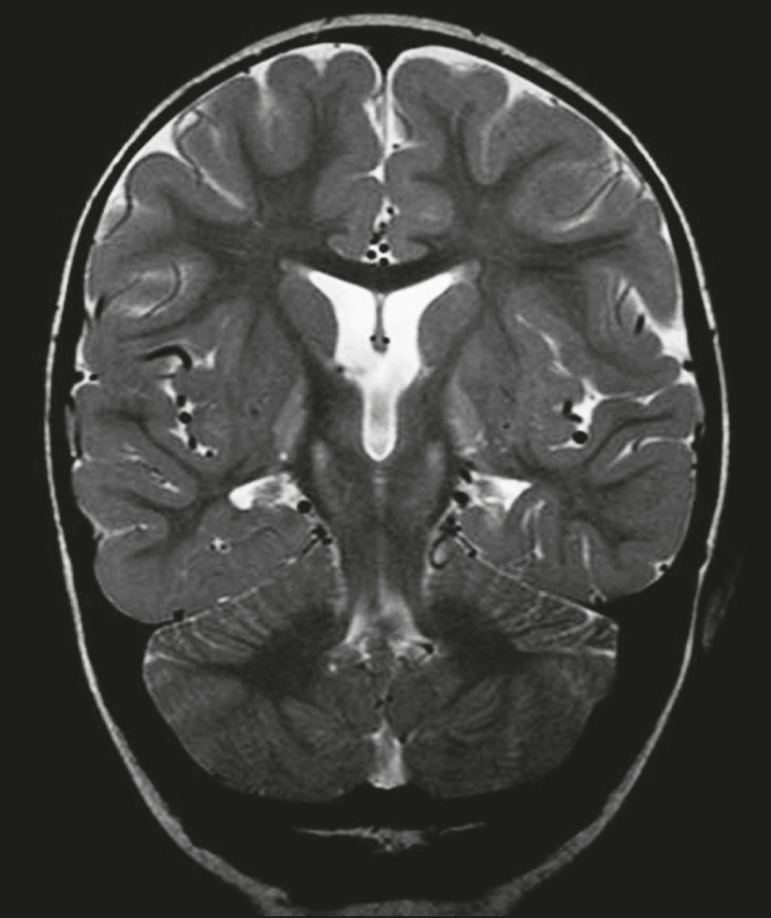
A 4-year-old female patient with a history of neonatal hyperbilirubinemia. Coronal T2-weighted MRI sequence showing a hyperintense signal in the globus pallidus, as well as, to a lesser extent, in the subthalamic, mesencephalic, and hippocampal regions (with reduced hippocampal volume).
